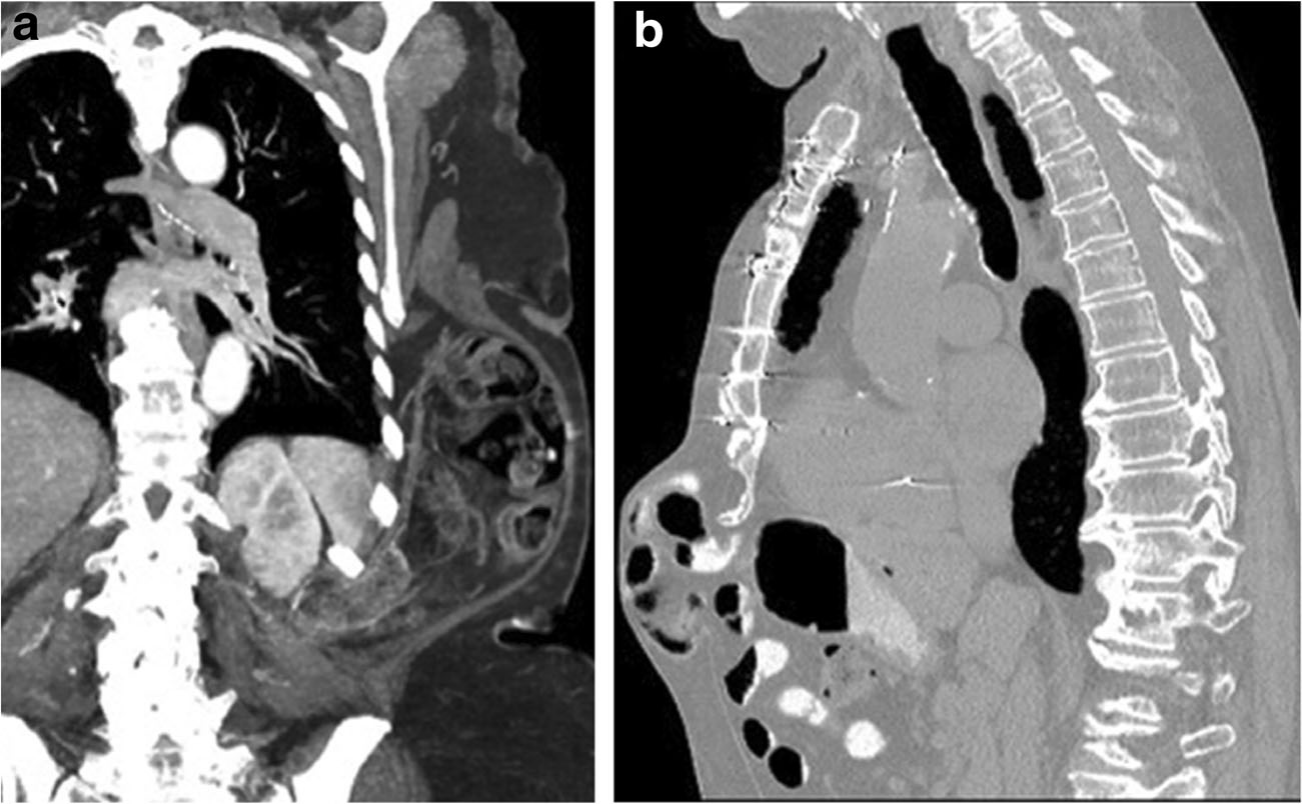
a An 80-year-old female being evaluated for transcatheter aortic valve replacement for severe aortic stenosis. Coronal maximum intensity projection image from a contrast-enhanced CT demonstrates a large left lateral abdominal wall hernia that originates at the lumbar triangle. The hernia sac is wide and contains small bowel, large bowel and omentum. It extends into the subcutaneous fascia of the thorax up to the level of the left 6th rib. b A 77-year-old male with lung cancer treated with stereotactic radiation therapy. Sagittal CECT obtained for surveillance demonstrates a large anterior abdominal wall hernia with subcutaneous extension into the pre-xiphoid portion of the anterior thorax
